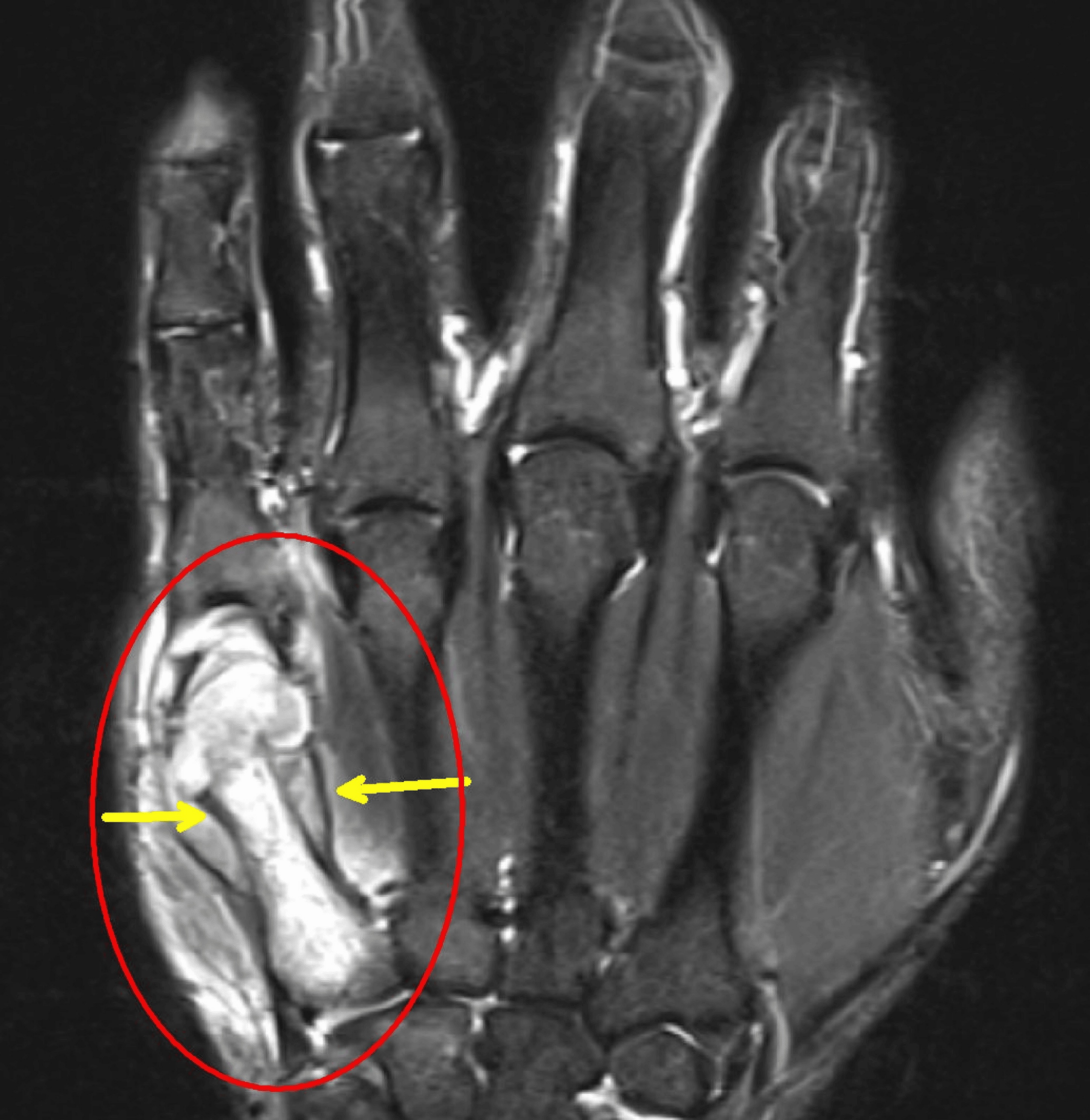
MRI of the right hand shows osteomyelitis in the fifth metacarpal bone (red circle) and an abscess lifting the periosteum (the two dark lines medial and lateral to the shaft, shown by the yellow arrows
